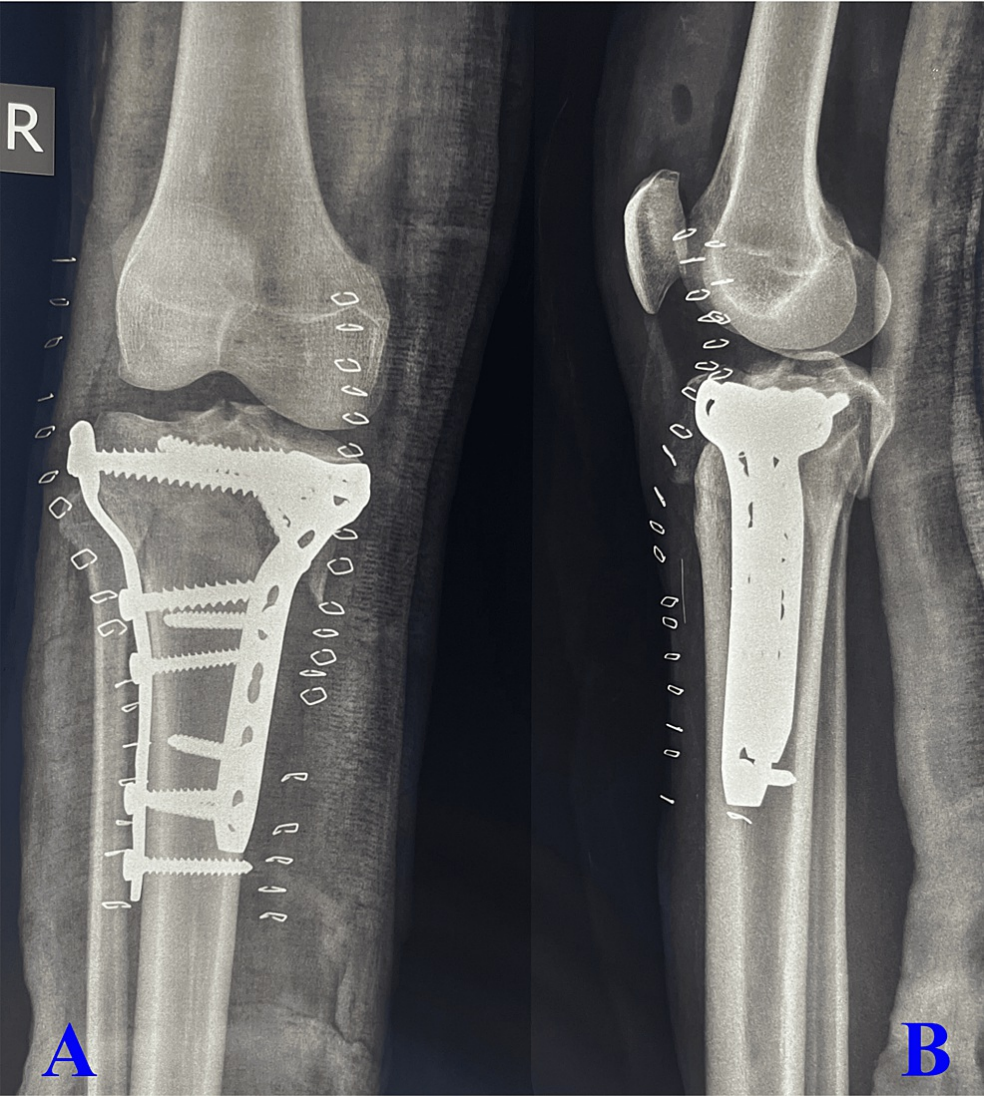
Postoperative X-rays showing the anteroposterior (A) and lateral (B) views A: The anteroposterior view shows adequate reduction of both the column with lateral and medial column plate (3.5 mm pre-contoured proximal lateral tibial plate and 3.5 mm T buttress plate for medial column). B: The lateral view displays adequate reduction and stabilization of the posterior segment of the proximal tibia (T buttress plate).
